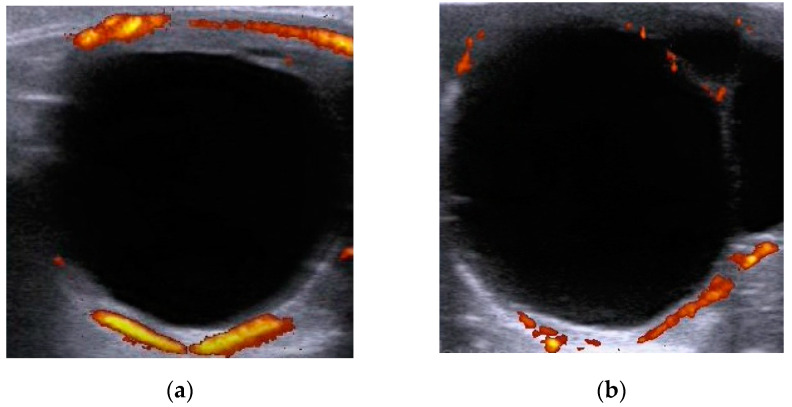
Representative image of preovulatory follicles, obtained at the time of ovulation induction, using Power Doppler ultrasound. (a) Blood flow follicle of a young mare; (b) Blood flow follicle of an aged mare.
